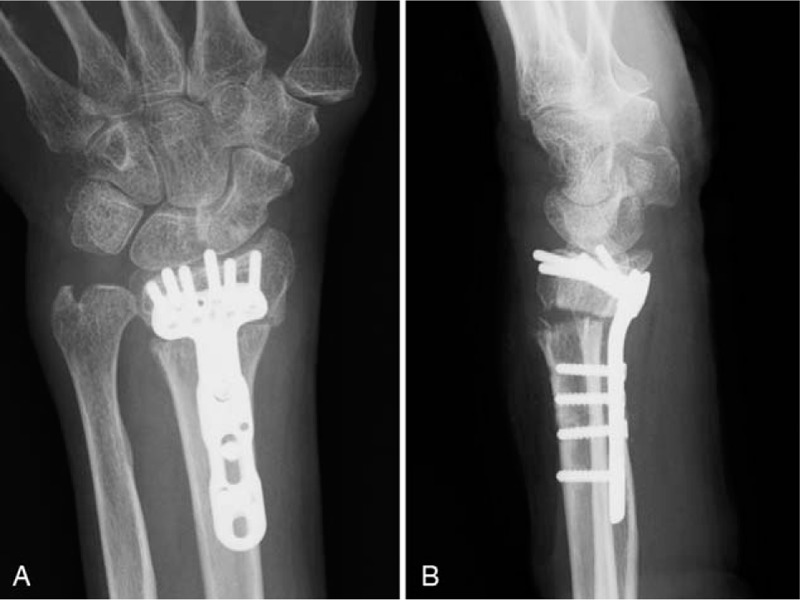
Postoperative radiographs of the left wrist. Anteroposterior (A) and lateral (B) views. The dorsal angulation of the distal radius is corrected and fixed with a volar locking plate.
